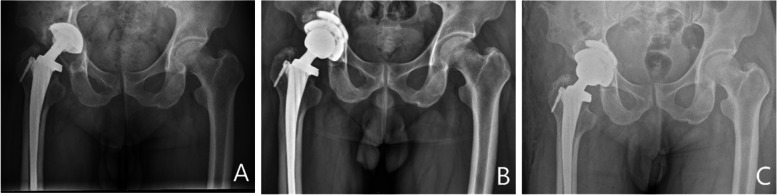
Five years after primary total hip arthroplasty, a 47-year-old man underwent revision surgery using a three-dimensional printed trabecular titanium acetabular cup and augment due to aseptic loosening. A Pre-operative radiograph showing a large acetabular defec. B Radiograph obtained at 1 day postoperatively. C Radiograph obtained at 25 months postoperatively showing the absence of a radiolucent line
